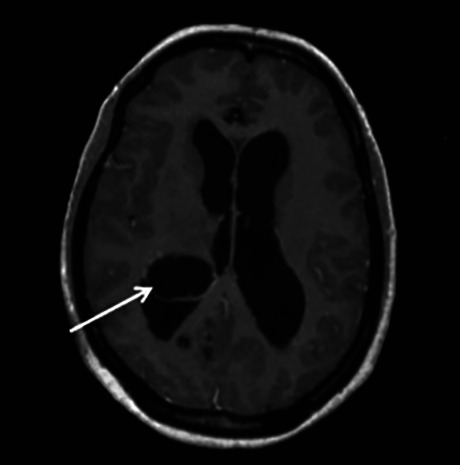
MRI intraventricular cysticercosis.
